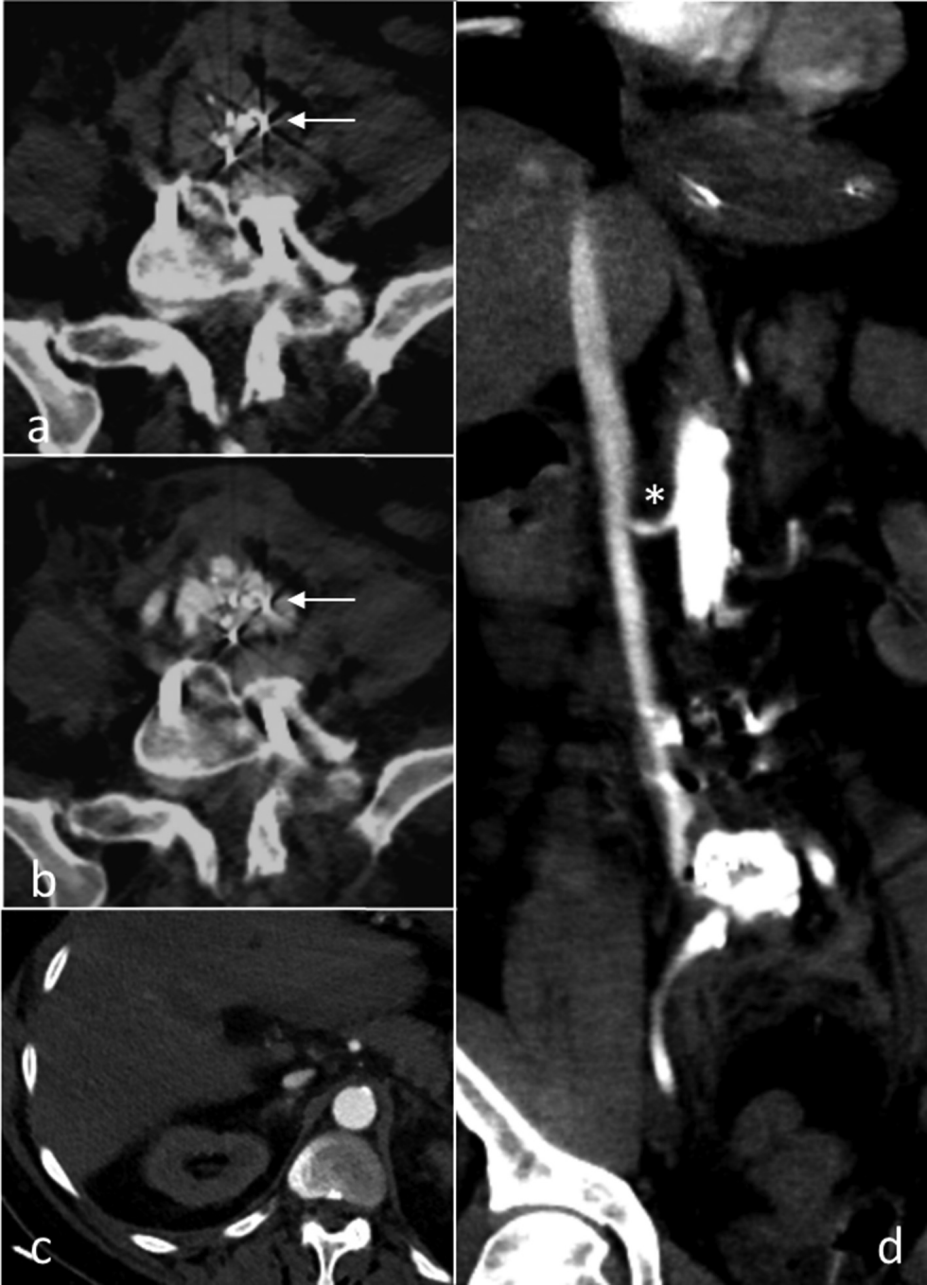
CT scan immediately after conclusion of surgery in prone position. Non-contrast-enhanced (a) and contrast-enhanced (b) scans show the dislodged transforaminal lumbar interbody fusion spacer in the prevertebral space (arrows). Significant vascular trauma has led to the formation of a pseudoaneurysm with incorporation of the spacer. Early arterial contrast phase documents extensive contrast within the inferior vena cava (c, d) up to the diaphragm indicating an arteriovenous fistula. Please note that the right renal artery (star) is merely located in close proximity to the inferior vena cava (d) without further vessel anomaly.
